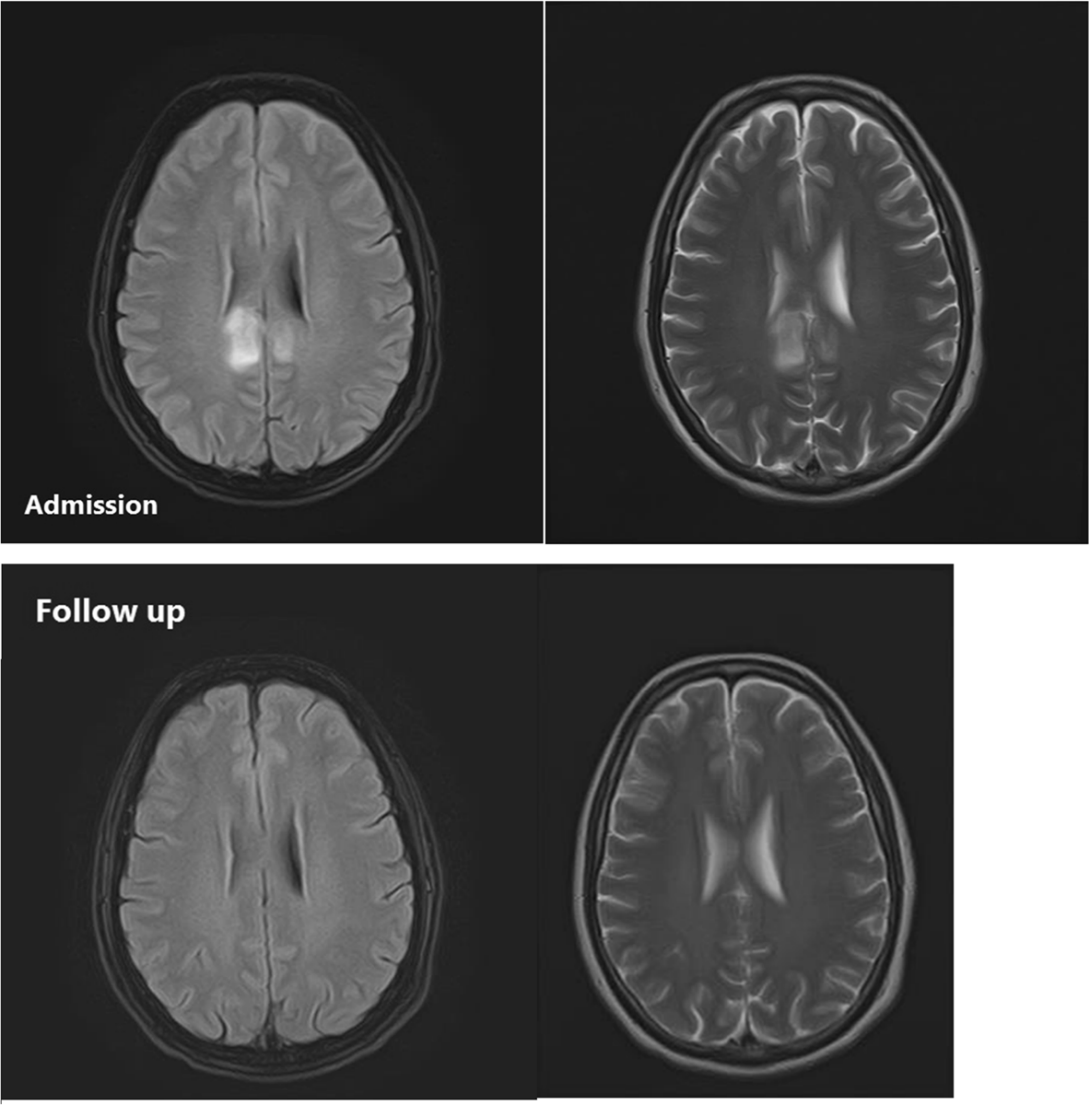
(Case 3): Admission MRI: MRI shows symmetric involvement of the parietal lobes in the parasagittal region. Gray matter, gray white matter junction, and subcortical white matter are affected. The lesions were bright on T2-weighted and FLAIR images and hypointense on T1-weighted images. Mild to moderate edema is associated with these lesions. No evidence of diffusion restriction is noted in mentioned areas. Follow-up MRI: Complete recovery of the lesions are seen in the repeat MRI after chelation therapy
